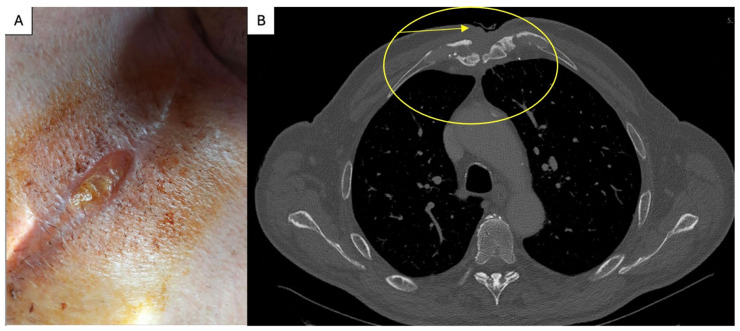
(A) SCF at second presentation; (B) Axial chest CT showing manubriosternal irregularity with a hypodense tract extending to the skin, consistent with fistulous communication and low-grade inflammation (arrow); the circle represents the area at the anterior chest wall were the SCF is located.
